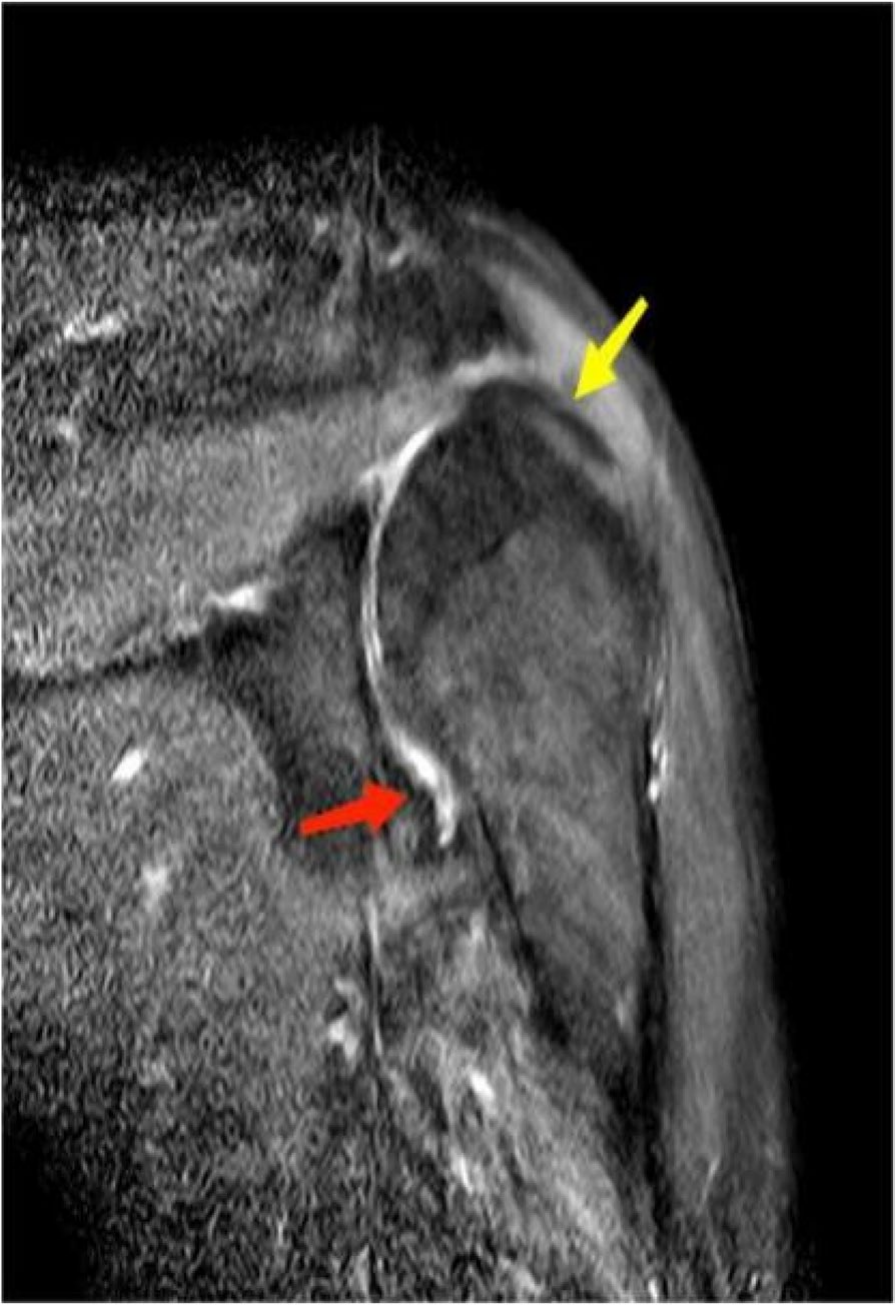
MRI image, Coronal T2WI. Male, 69 years old
